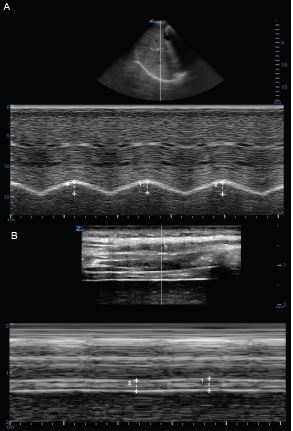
Diaphragmatic ultrasound. A) Diaphragmatic excursion on M-mode in subcostal view at the mid-clavicular line. B) Diaphragmatic thickness on M-mode at the mid-axillary line.
